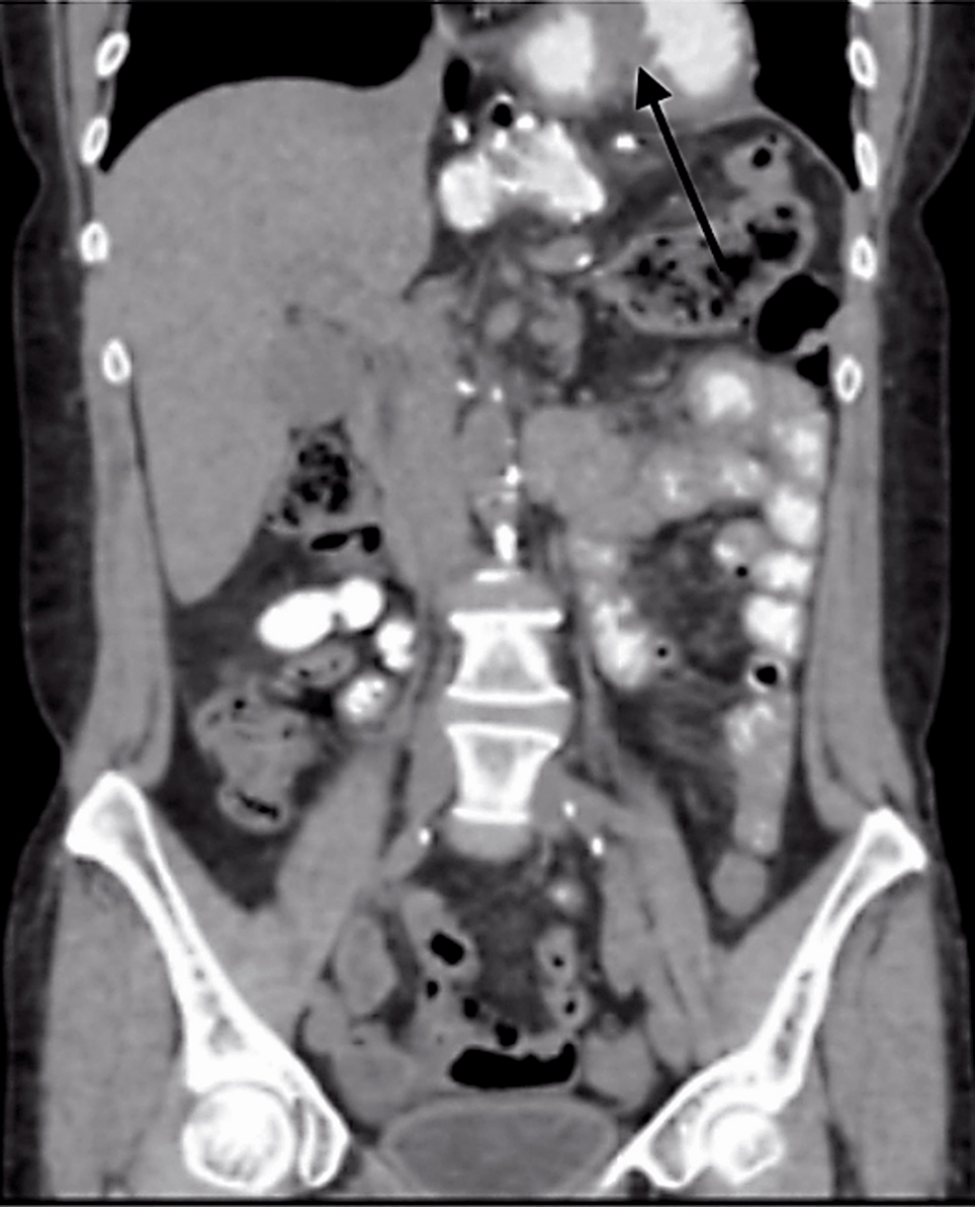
CT scan showing herniated jejunum above the diaphragm (marked with an arrow).
